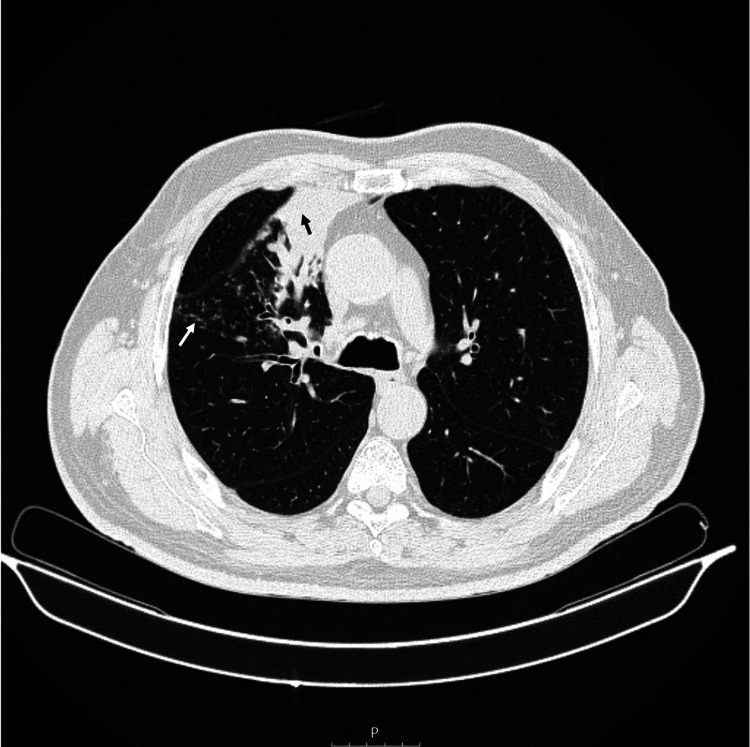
Initial chest computed tomography Chest computed tomography showed an area of parenchymal consolidation in the anterior segment of the upper right lobe of the lung (black arrow) with centrilobular micronodules in a tree-in-bud morphology (white arrow).
